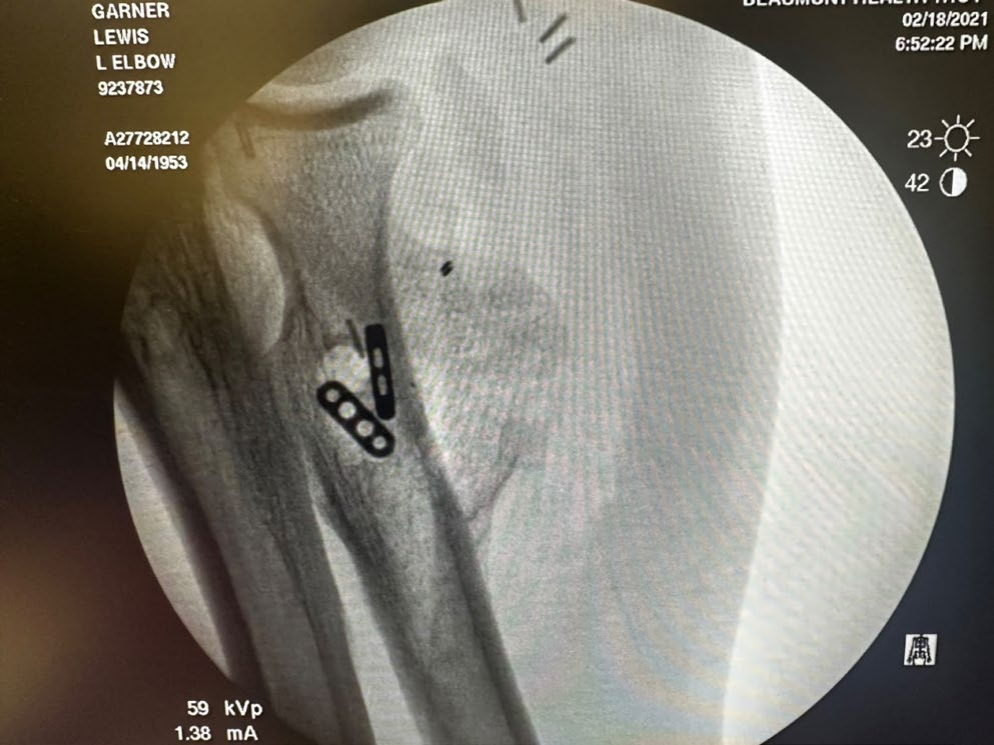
Intraoperative radiograph showing perfect alignment of secondary and primary fibertak stitch with significant decrease of heterotopic ossification.
